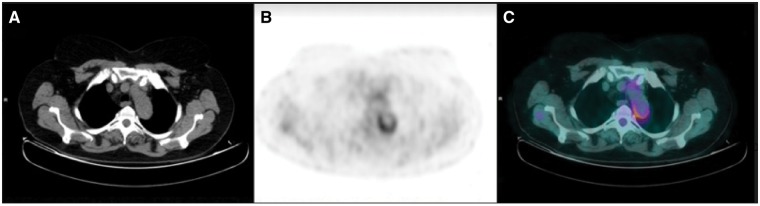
^18^F-Fluorodeoxyglucose PET/CT images FDG PET/CT images showed an area of increased radiopharmaceutical uptake in the aortic arch walls. (A) Axial CT slice. (B) Axial PET slice. (C) Axial fused images. FDG: fluorodeoxyglucose.
